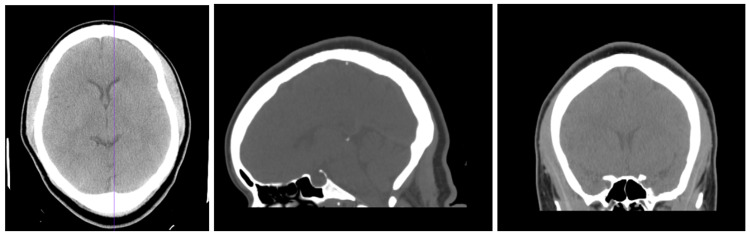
A noncontrast computed tomography of the patient’s head taken on November 9, 2020, one day prior to her discharge Image 1: Axial view of the patient's brain on computed tomography Image 2: Sagittal view of the patient's brain on computed tomography Image 3: Coronal view of the patient's brain on computed tomography
